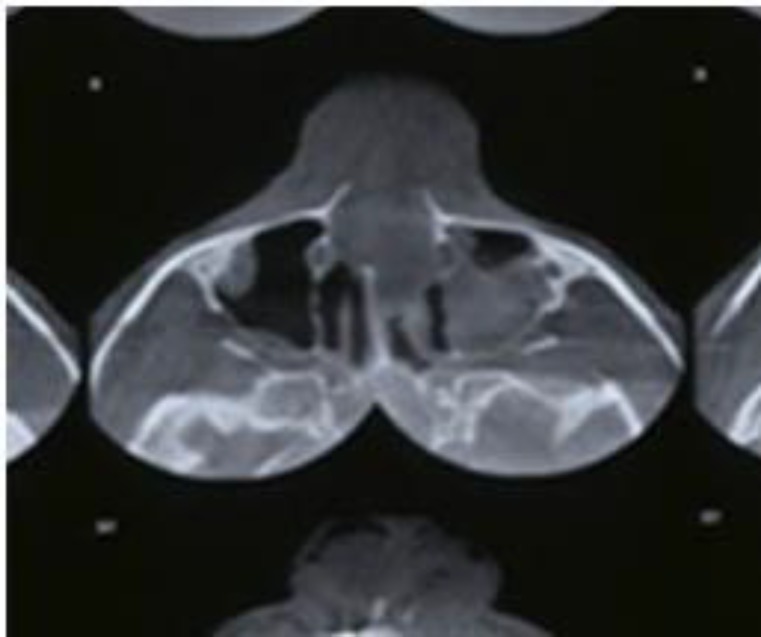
Postoperative CBCT of maxillary orthognathic patient with delayed nose bleeding, with origin at lateral maxillary wall
